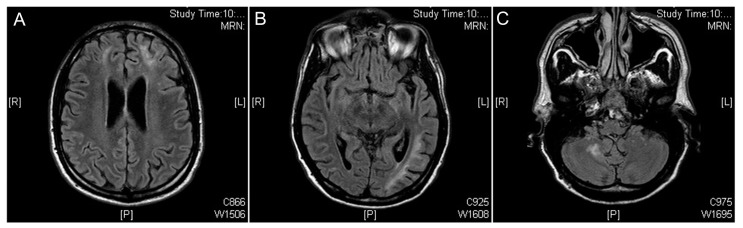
Case 1, Neurosyphilis. Magnetic resonance imaging (MRI) of the brain with gadolinium showing the presence of multiple hyperintense lesions in long repetition time (TR) sequences localized bilaterally in frontal hemispheres (A); in temporal, parietal and occipital left hemispheres (B) and in the right cerebellum peduncle (C). MRN: magnetic resonance neurography; R: right; L: left; P: posterior.
